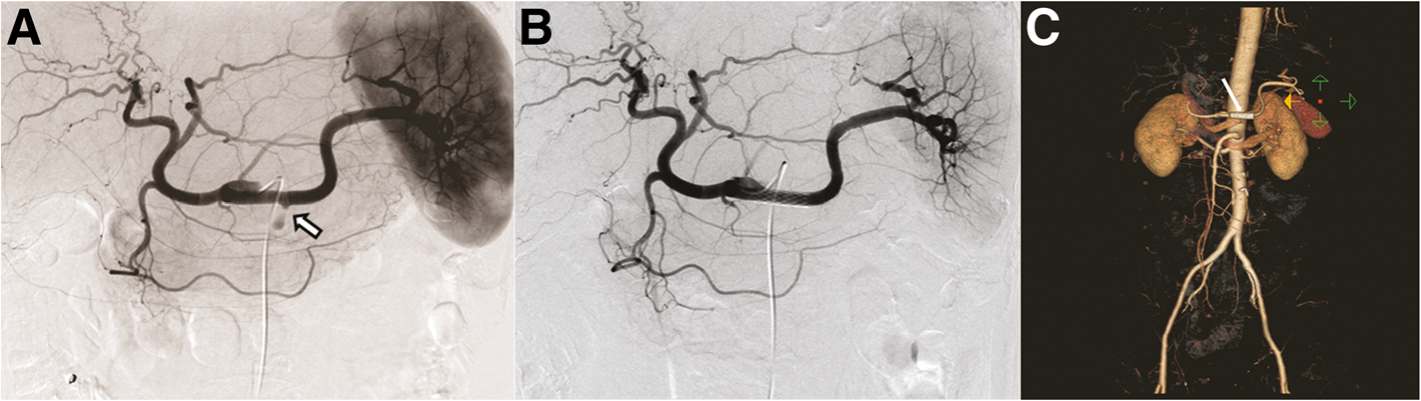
Angiographic finding and endovascular treatment. a Angiography of the splenic artery revealed a 5-mm saccular aneurysm of the splenic artery (arrow). b The splenic artery aneurysm after implantation of a 28-mm Jostent. c On a contrast-enhanced abdominal angiography CT scan obtained 10 days after the procedure, the splenic artery aneurysm was completely excluded (Stent, arrow)
